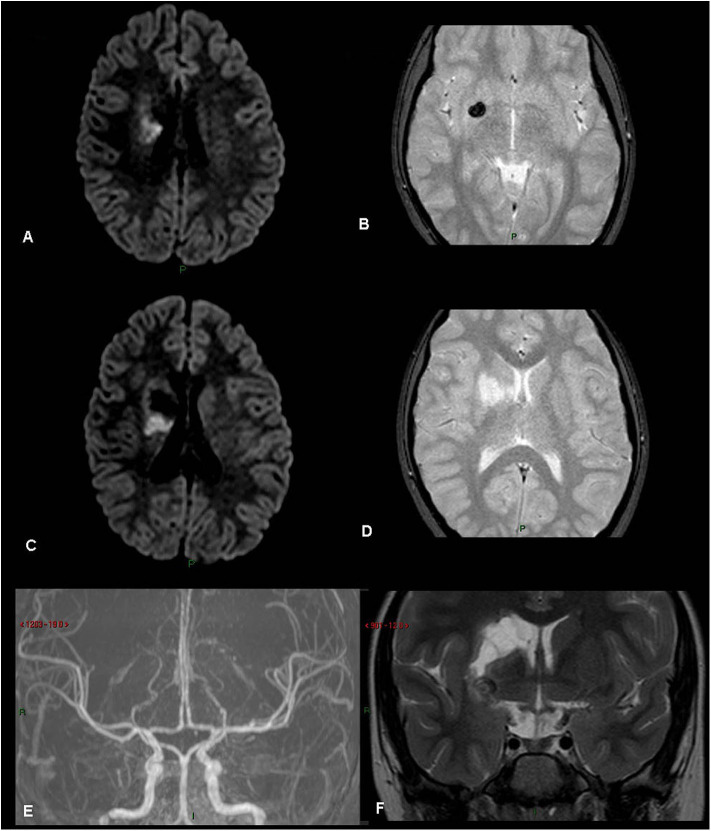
Rt basal ganglia old infarct with hemorrhage. (patient 21, Table 1) (A) FLAIR. (B) Gradient Echo T2. (C) FLAIR. (D) Gradient Echo T2. (E) MRA TOS. (F) Coronal T2. Old right basal ganglia infarct with evidence of hemosiderin, old hemorrhage.
